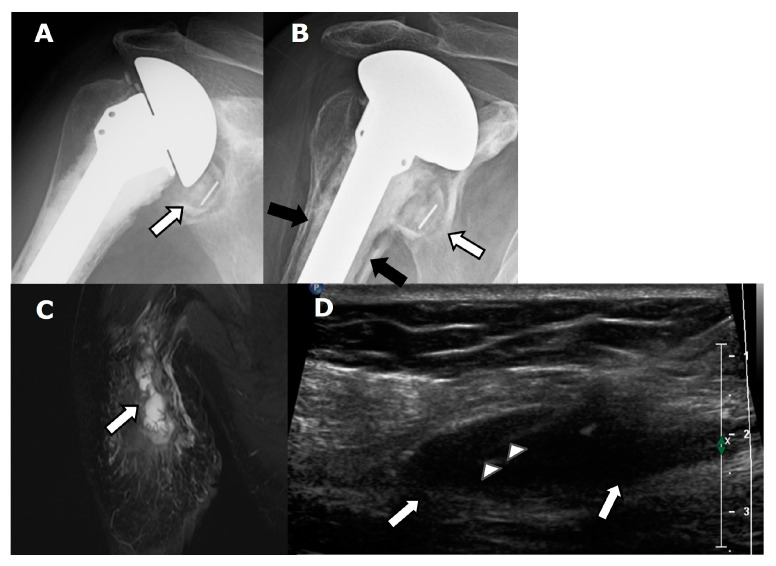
Patient with ATSA and periprosthetic joint infection in a patient with shoulder pain. (A). Frontal radiograph shows an ATSA with a small amount of lucency (arrow) around the glenoid component. (B). Frontal radiograph obtained several months later shows worsening lucency (white arrow) along the glenoid component, with new lucency (black arrows) along the bone–cement interface of the humeral component. (C). Coronal T2-weighted, fat-saturated MR image shows lobulated high signal (arrow) throughout the shoulder musculature. (D). Long-axis ultrasound (US) image shows an ovoid soft tissue fluid collection/abscess (arrows), with needle (arrowheads) present during diagnostic aspiration.
